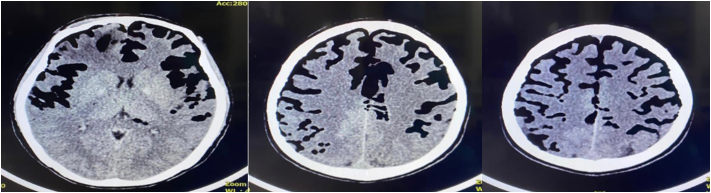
Serial CT scan of brain parenchym window at the second visit showing massive PNC in both frontoparietal lobes.
